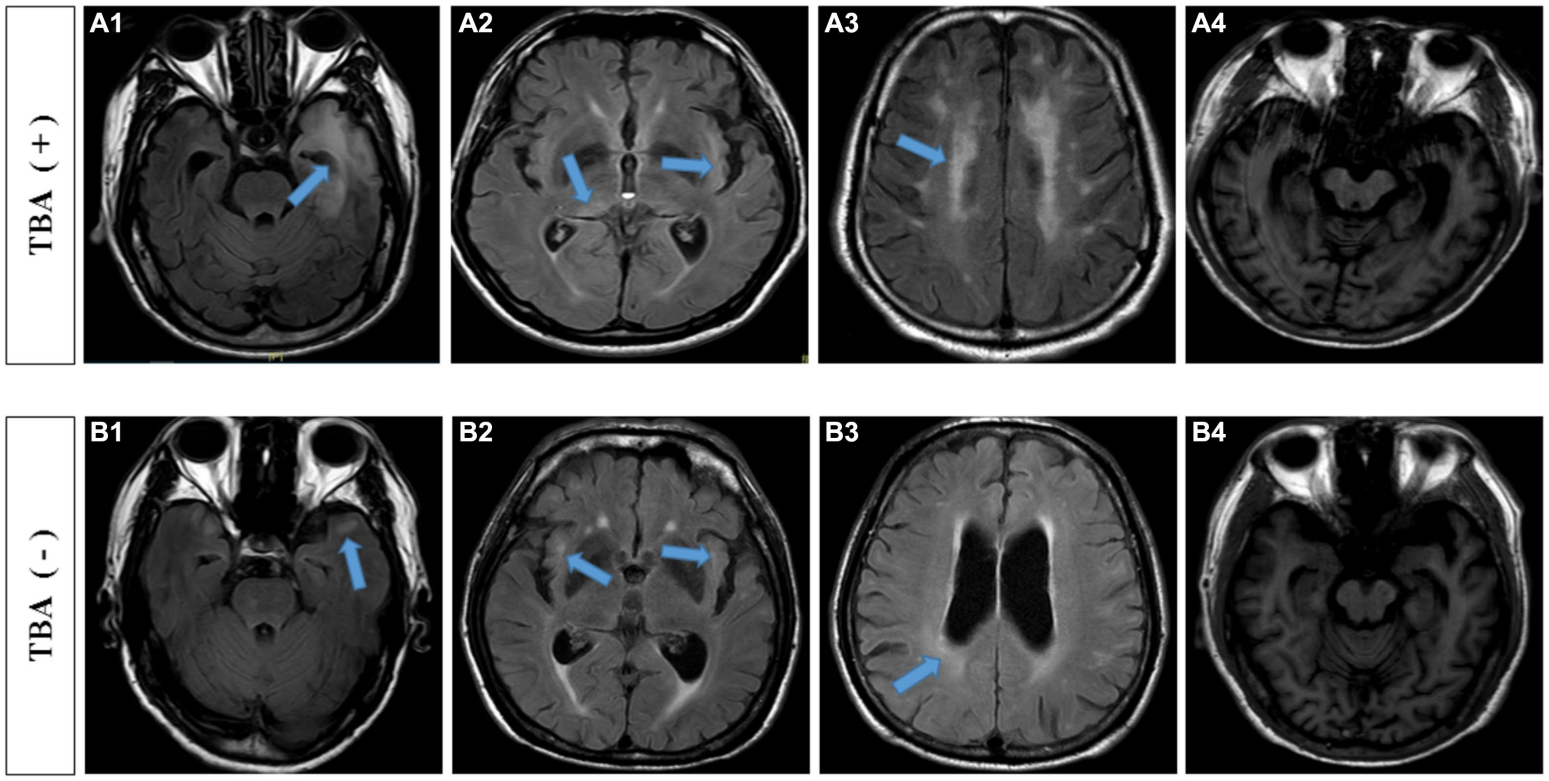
MRI findings of TBA-positive and-negative neurosyphilis patients. TBA-positive neurosyphilis patients: (A1,A2) parenchymal abnormalities in the frontotemporal and hippocampal regions on the cranial MRI images. (A3) white matter lesions, (A4) diffuse cerebral atrophy and hippocampal atrophy. TBA-negative neurosyphilis patients: (B1,B2) parenchymal abnormalities in the frontotemporal and hippocampal regions on the cranial MRI images. (B3) white matter lesions, (B4) diffuse cerebral atrophy and hippocampal atrophy. MRI, magnetic resonance image; TBA, tissue-based assay.
